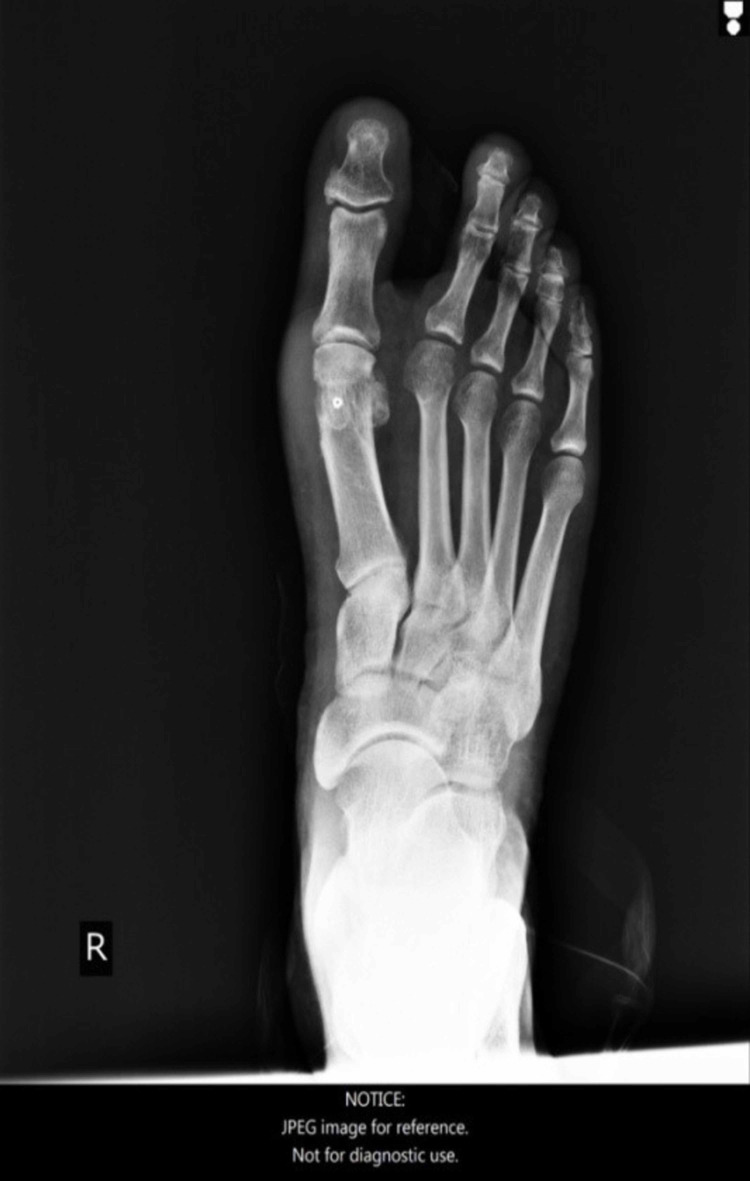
Foot X-ray, anteroposterior view, 27 months post-operatively.
